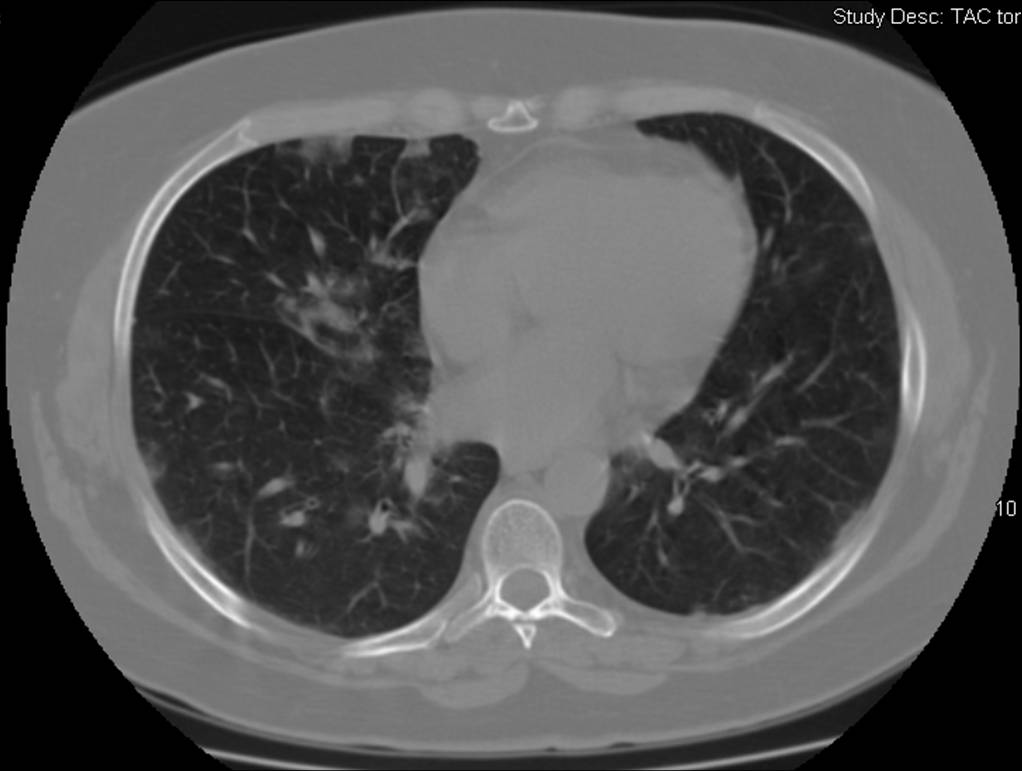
Chest CT scan shows ill-defined ground-glass opacities with thickened interlobular septa and some peripheral and central ill-defined nodules prevalent at the base in the right lung and diffusely in left lung.
